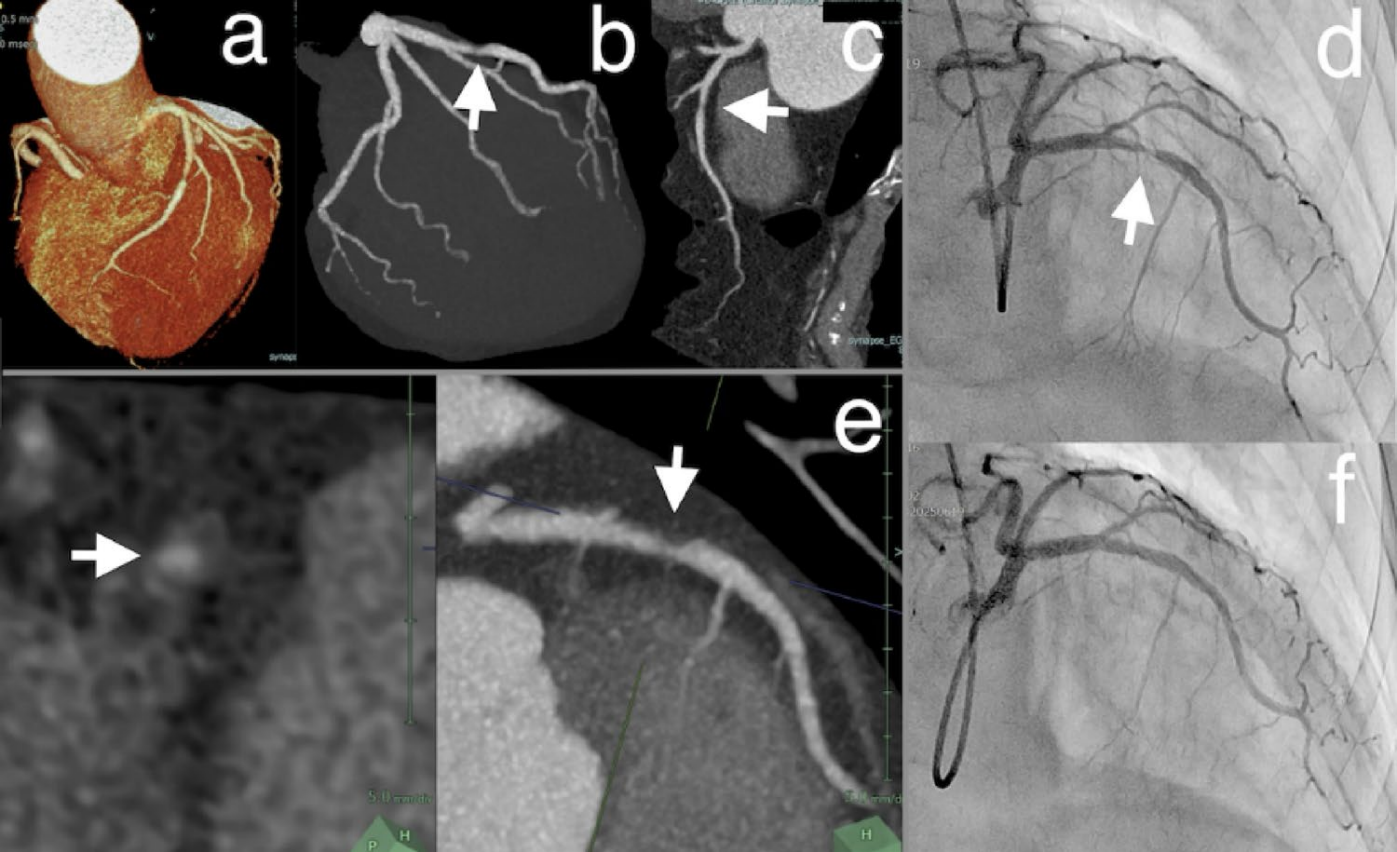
Cardiac CT and coronary angiography findings in a patient with a left anterior descending artery lesion. (a) A volume-rendered reconstruction of the coronary arteries demonstrates the overall anatomy. (b) A full-volume maximal intensity projection reveals a stenotic lesion in the left anterior descending artery. (c) A curved multiplanar reformation provides a detailed view of the lesion characteristics, though its 2D projection does not offer an accurate representation of the original 3D morphology. (d) Coronary angiography confirms severe stenosis in the left anterior descending artery. (e) Thin-slab MIP demonstrates a similar image to the coronary angiography, and the short-axial view reveals an eccentric low-density plaque. (f) Post-intervention coronary angiography shows successful placement of a drug-eluting stent using a slender 5-Fr guiding catheter. Arrows indicate the stenotic lesion. *2D* two-dimensional, *3D* three-dimensional, *CT* computed tomography, *Fr* French, *MIP* maximum intensity projection
